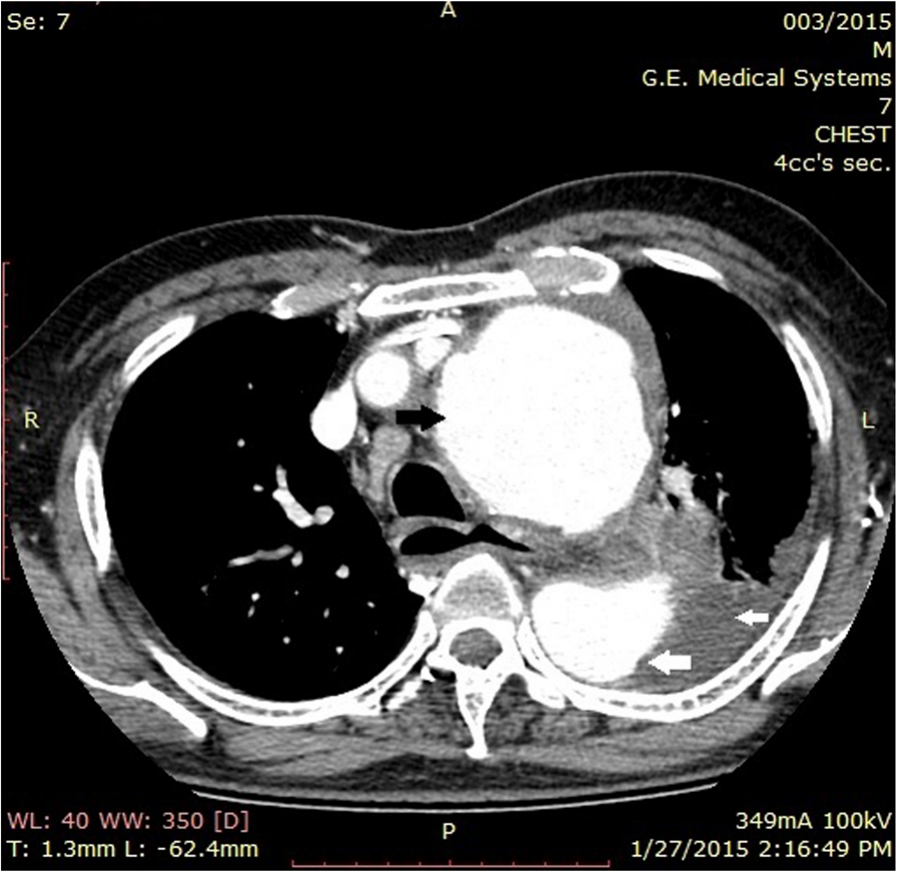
Computed tomography aortography showing ascending (black arrow) and descending (large white arrow) aortic aneurysm with left-sided pleural effusion (small white arrow)
